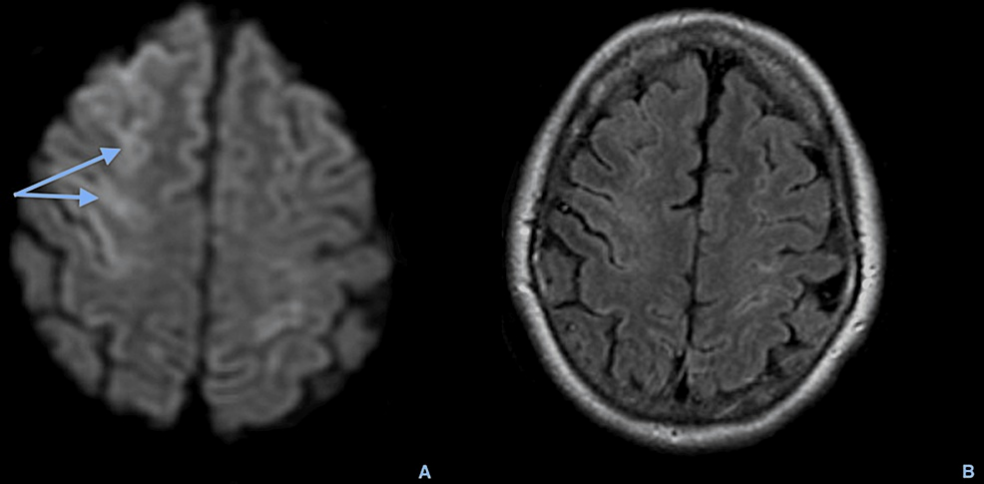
Axial brain magnetic resonance imaging, the first 24 hours after the event, showing on DWI a gyroscopic hypersignal in multiple cortical areas on the right frontal high convexity, consistent with a predominant cortical ischemic lesion (double blue arrow on A), still without translation on FLAIR (B) as expected in these lesions with a short time of onset
